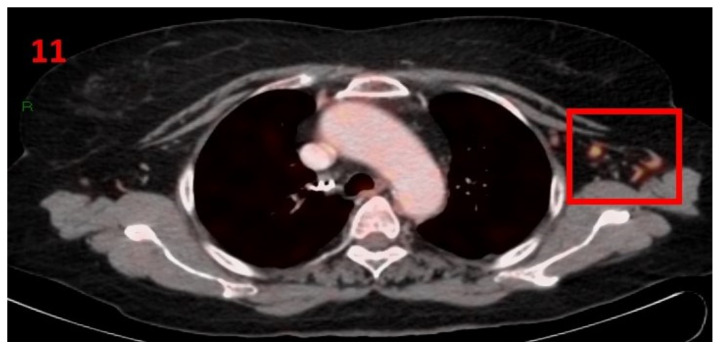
[18F]FDG PET-CT showing hypermetabolic uptake in the 11th patient with lymphadenopathy in the left axillary region (red square).
